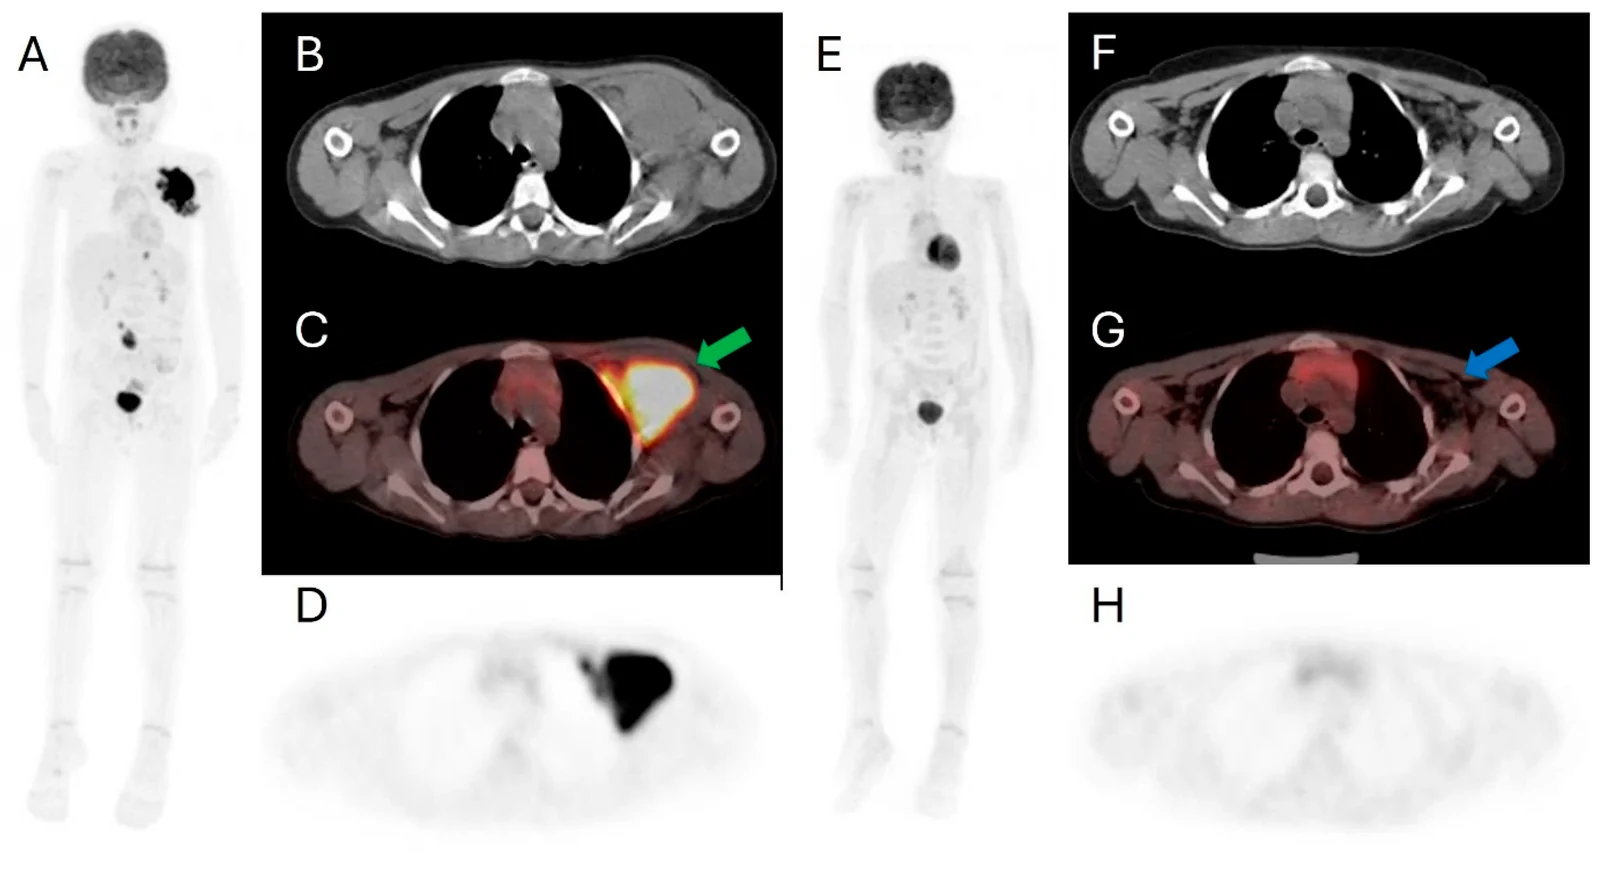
A 6-year-old boy with stage III pediatric Burkitt lymphoma involving the left axilla. (A) Baseline whole-body MIP showing an intensely hypermetabolic left axillary mass, with additional foci below the diaphragm (abdominal/pelvic region) consistent with subdiaphragmatic nodal involvement and the patient’s stage III classification. (B) Baseline axial CT showing the corresponding left axillary soft-tissue mass. (C) Baseline axial fused PET/CT confirming intense FDG uptake within the mass (SUVmax 13.8; green arrow). (D) Baseline axial PET image showing the corresponding hypermetabolic focus. (E) End-of-treatment whole-body MIP showing resolution of the previously FDG-avid mass. (F) End-of-treatment axial CT showing near-complete resolution of the soft-tissue mass. (G) End-of-treatment axial fused PET/CT showing only background-level activity at the site of the prior lesion (blue arrow), corresponding to a Deauville score of 2. (H) End-of-treatment axial PET image showing no residual abnormal uptake. Baseline MTV, TLG, and SUVmax were 82.2 cm^3^, 723.3 g, and 13.8, respectively; corresponding end-of-treatment values were 15.9 cm^3^, 10.4 g, and 1.1, representing a 92.0% reduction in SUVmax, consistent with the study cutoff (≥73.6%) for favorable metabolic response.
